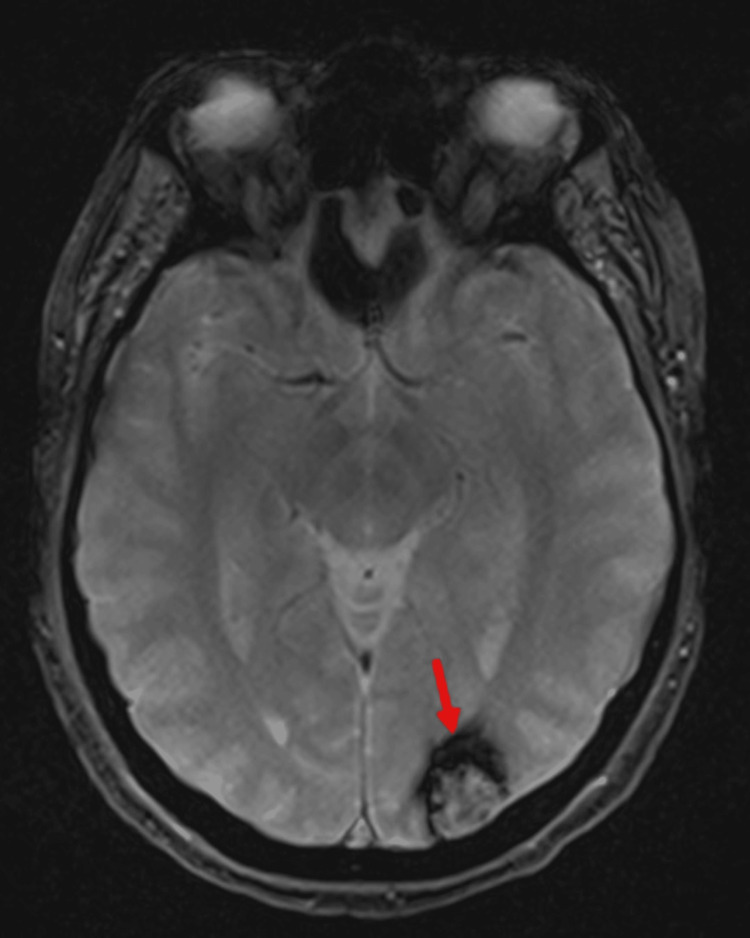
MRI of the brain Axial T2*/GRE MRI demonstrating a rounded left occipital cortico-subcortical lesion measuring 17 × 16.5 × 16 mm, with heterogeneous signal intensity and a peripheral hypointense hemosiderin rim producing a blooming artifact, consistent with a Zabramski type II cavernous malformation (red arrow). No additional structural abnormalities are identified. MRI: magnetic resonance imaging, GRE: gradient-recalled echo
